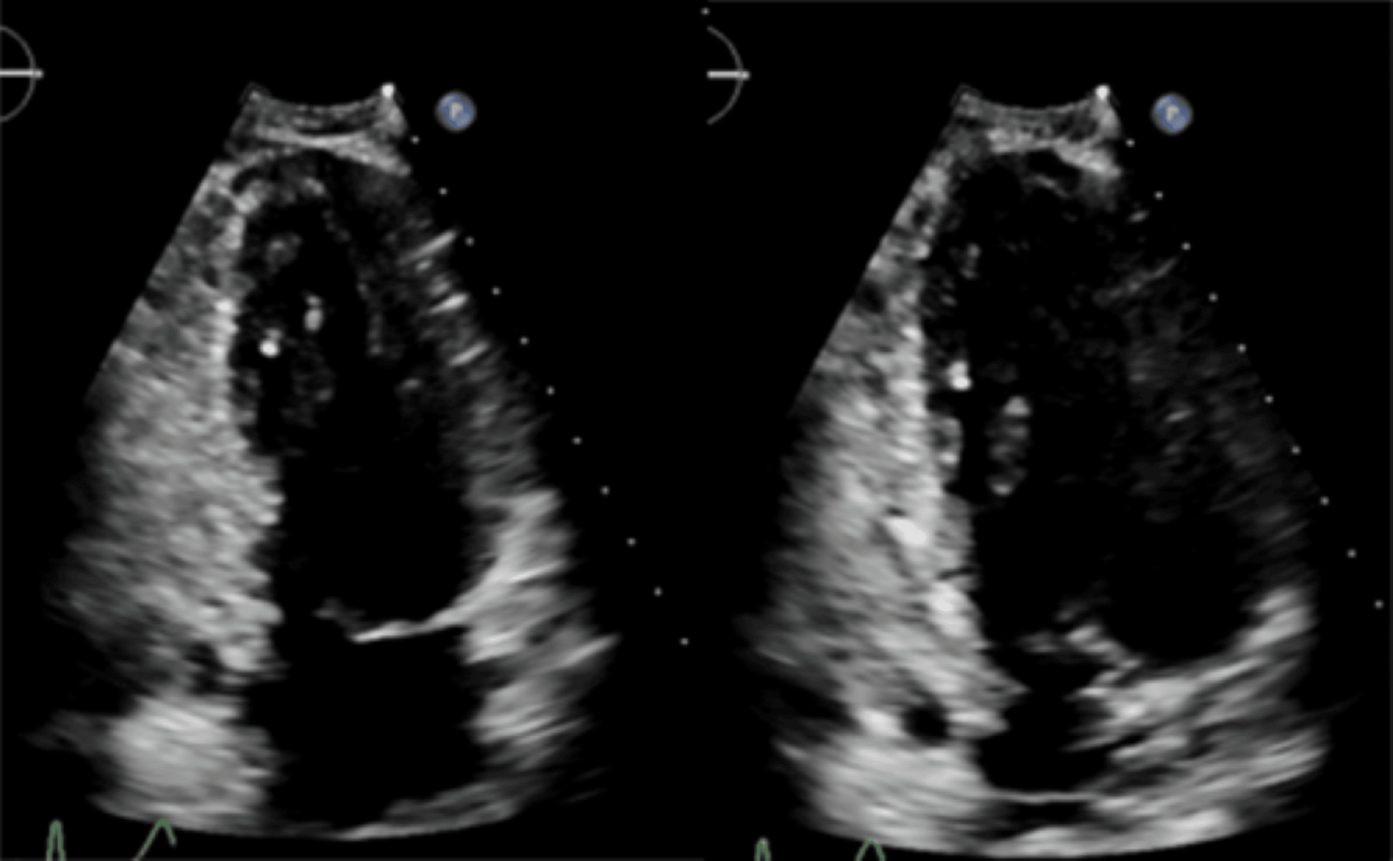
Transthoracic echocardiogram showing improved ejection fraction after resolution of sepsis
